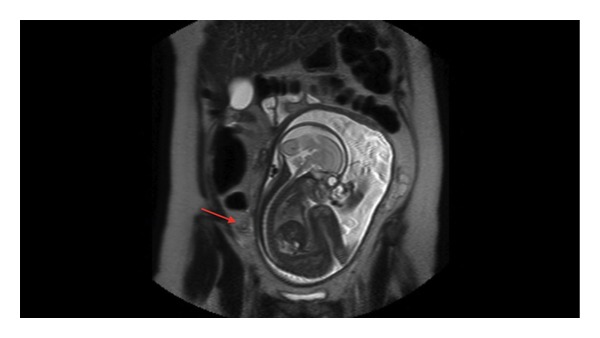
MRI T2 coronal view: free fluid and inflammatory changes around the appendix (arrow). Gravid uterus.
